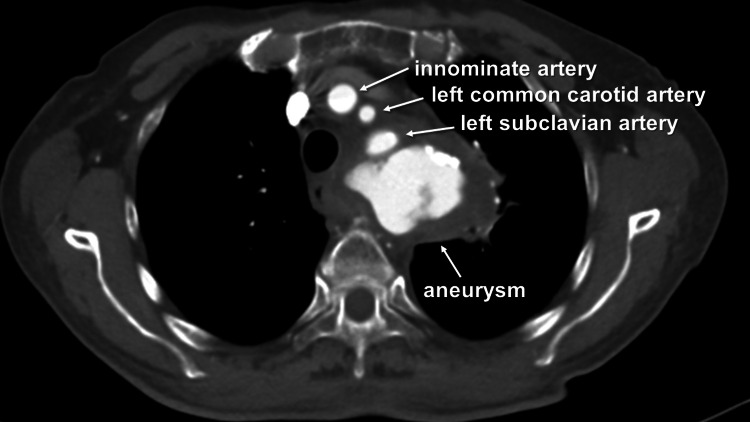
Preoperative contrast-enhanced computed tomography revealing a distal arch aneurysm
